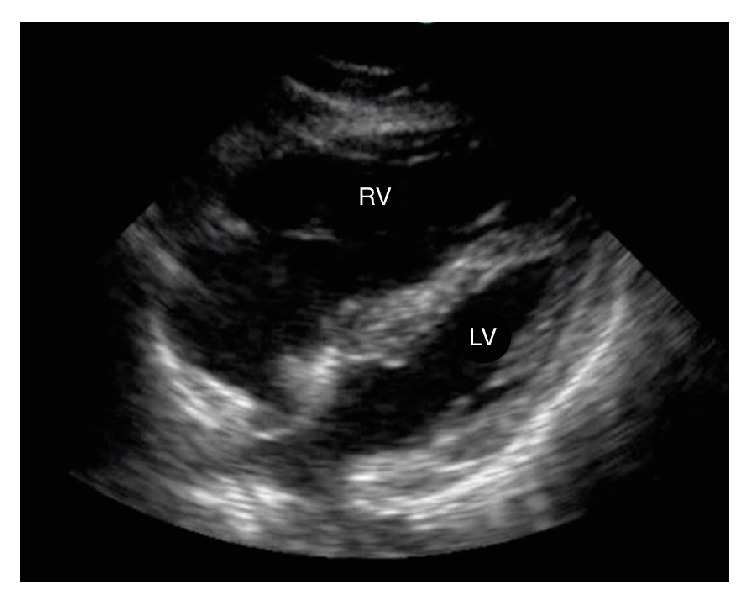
Cardiac ultrasound, subxiphoid view showing a dilated right ventricle (RV).
